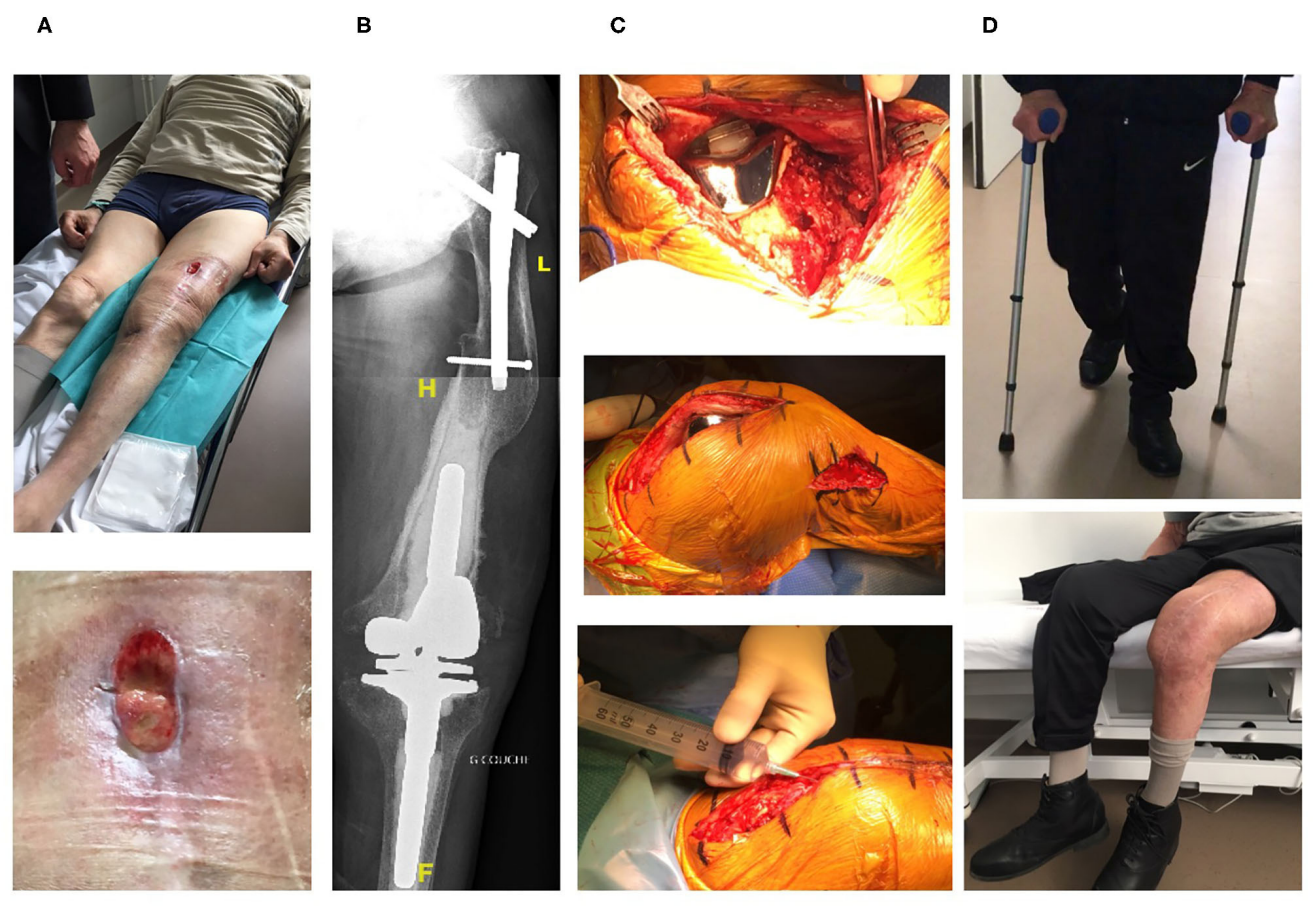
The first patient is an 80-year-old man with past history of Parkinson disease, cardiac arrhythmia, hypertension, right hip prosthetic joint, and left hip fracture treated with osteosynthesis. A left knee prosthetic joint arthroplasty was performed in 2004. In 2014, the patient developed signs of septic arthritis of the left knee due to a methicillin-susceptible *S. aureus*. A one-stage exchange was performed with reimplantation of a hinged knee prosthesis with long cemented stem. The patient received intravenous daptomycin and rifampin orally, followed by cotrimoxazole and clindamycin for a total duration of 3 months. Unfortunately, a relapse occurred in April 2015 with persistence of the *S. aureus*, and pristinamycin (a streptogramin A+B antibiotic available in France) was prescribed as suppressive antimicrobial therapy. Despite pristinamycin treatment, the patient developed signs of left septic arthritis with severe pain, bedridden and large anterior fistula with purulent discharge (A). X-ray revealed a complex orthopedic situation with radiological signs of prosthesis loosening of the femoral stem (B). Open DAIR was performed showing poor soft tissue condition with pus in contact to the implant and the personalized cocktail of phages was injected in joint just after closure (C). The patient improved quickly, but at 3 months, a mild discharge of synoviual fluid persisted (Figure 5). A new DAIR was performed, revealing a drastic improvement of the local conditions, with only mild signs of non-specific synovitis. Multiple samples were performed for bacterial culture, but no recurrency/superinfection was diagnosed (cultures remained sterile, specific *S. aureus* PCR was negative). Cefalexin was then prescribed as suppressive therapy, and the outcome was favorable after 2 years and half of follow-up with no sign of infection, a negative C-reactive protein, and a pain-free walking [(D), Supplementary Video 1].
